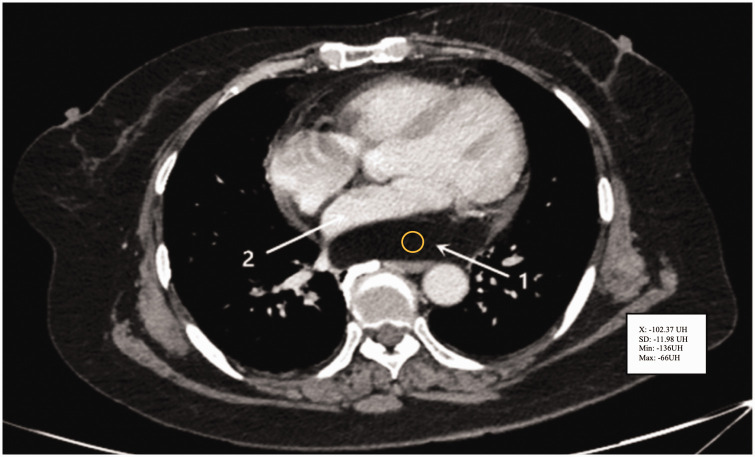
Axial cardiac computed tomography image showing a large low-density mass located on the posterior wall (1) of the left atrium (2) without enhancement, which suggests cardiac lipoma (used with permission).
